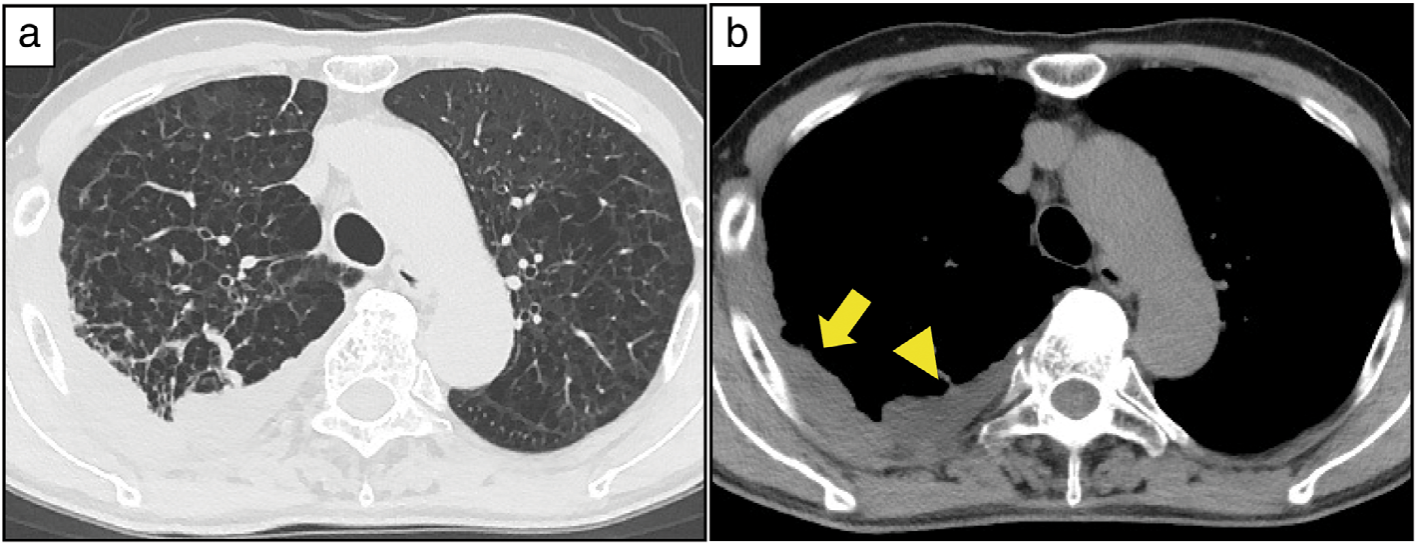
Preoperative computed tomography images. (a) Significant emphysema observed in the pulmonary window setting. (b) Right pleural thickening (yellow arrow) and effusion (yellow arrowhead) observed in the mediastinal window setting
